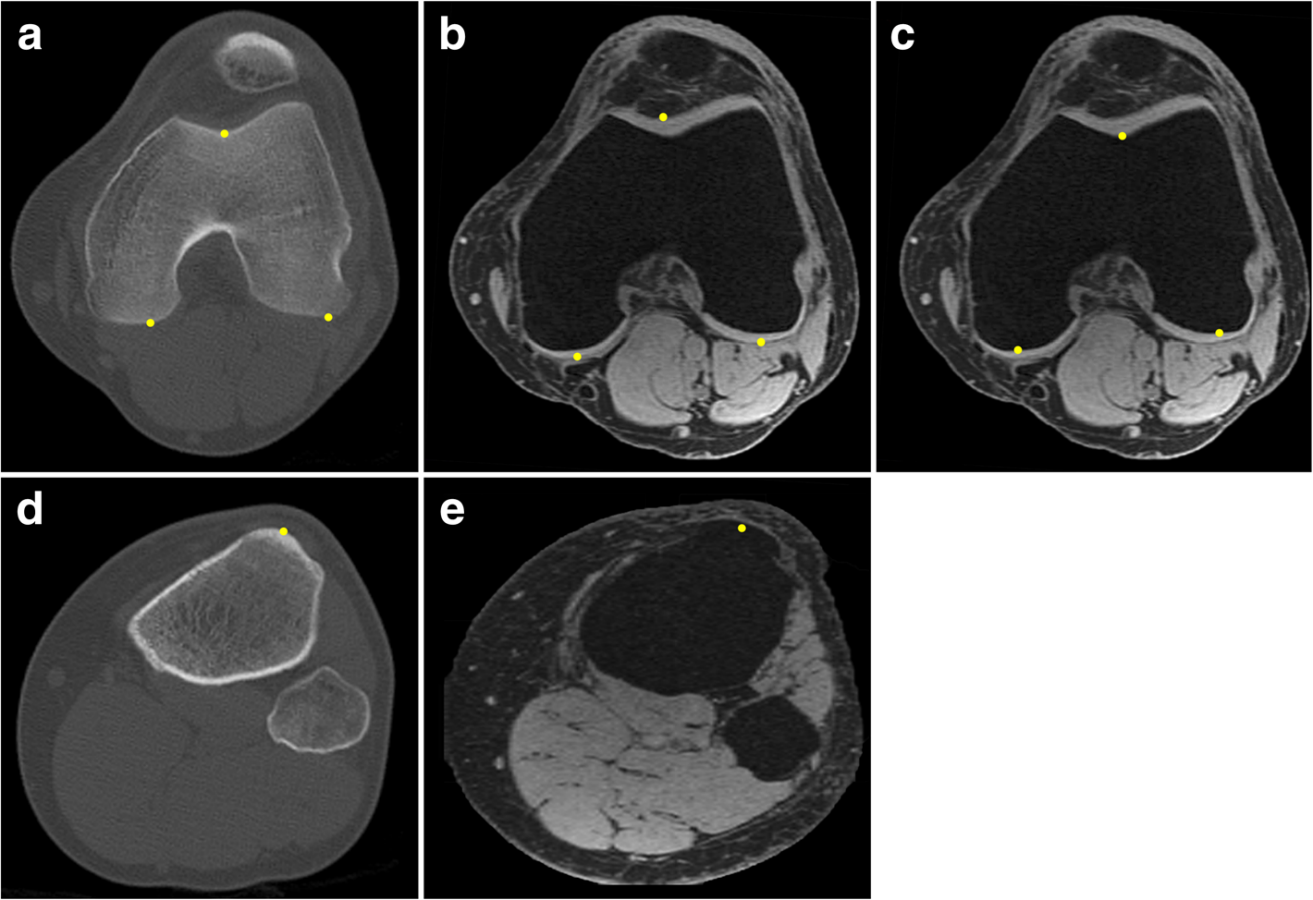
Markings of the anatomical landmarks for the measurement of the tibial tubercle-trochlear groove distance. The deepest point of the trochlear groove, the points of tangency of the posterior femoral condyles, and the most prominent point of the tibial tubercle are identified and marked. a CT of the distal femur. b MRI of the distal femur with cartilaginous landmarks. c MRI of the distal femur with osseous landmarks. d CT of the proximal tibia. e MRI of the proximal tibia
